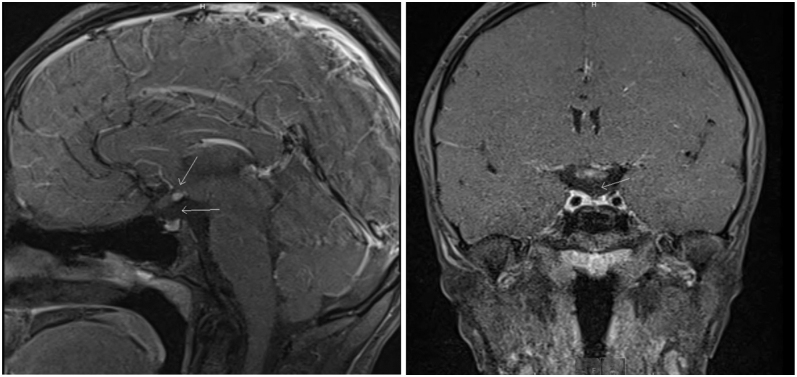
MRI demonstrating pituitary stalk interruption syndrome (PSIS). MRI demonstrating hypoplasia of the anterior pituitary with arrows denoting the absent infundibulum, findings compatible with PSIS.
